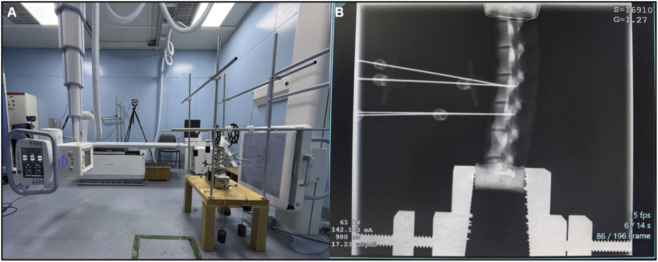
Dynamic X-ray System. (A) Dynamic X-ray system device; (B) DI-X1 image diagnostic processing software used for fluoroscopic image analysis.
